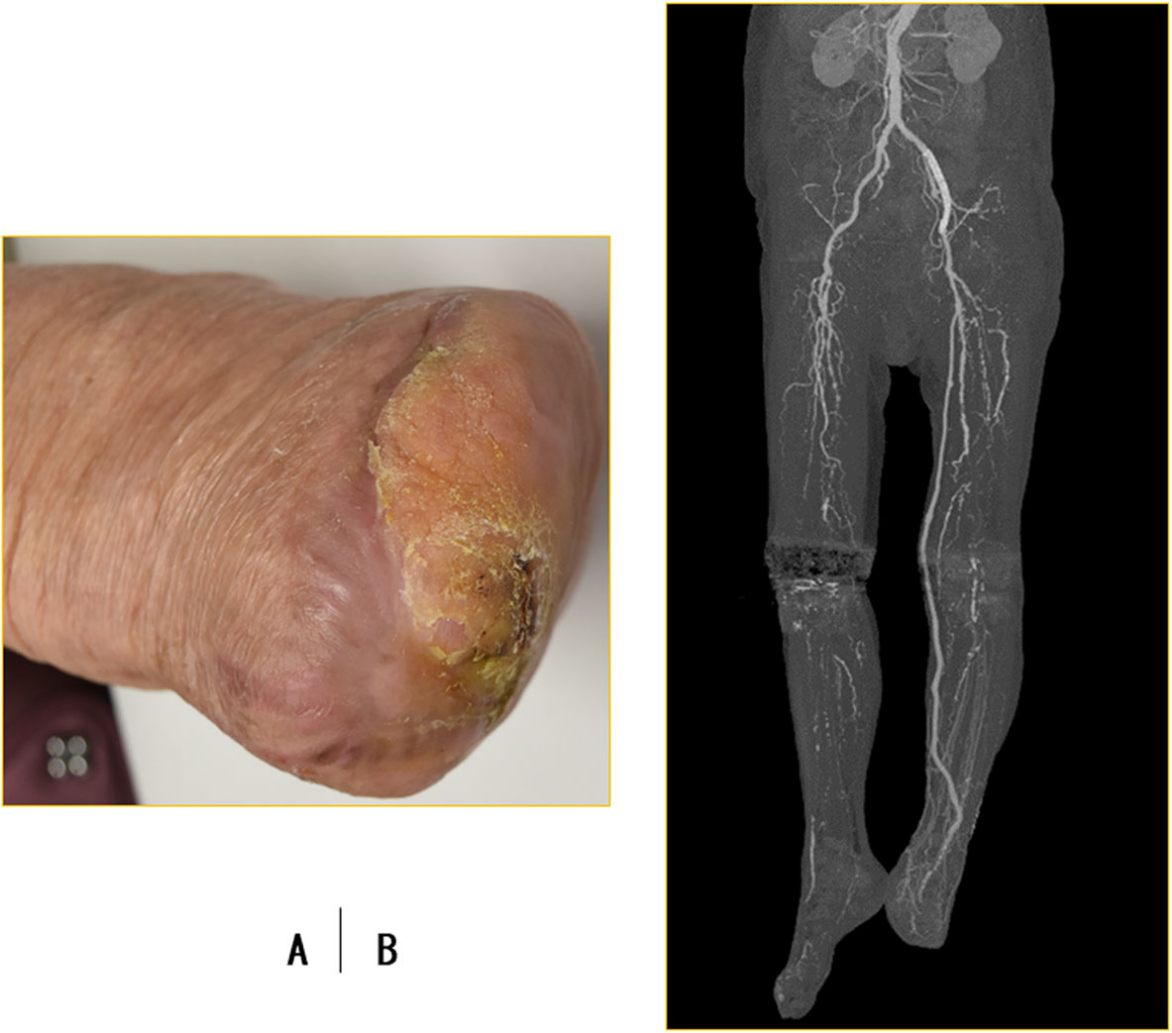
The stump of the amputated toes completely healed (a, 6 months after the bypass surgery). Follow-up contrast-enhanced computed tomography revealed that there were no complications, such as occlusion or aneurysm, of the varicose vein graft (b)
